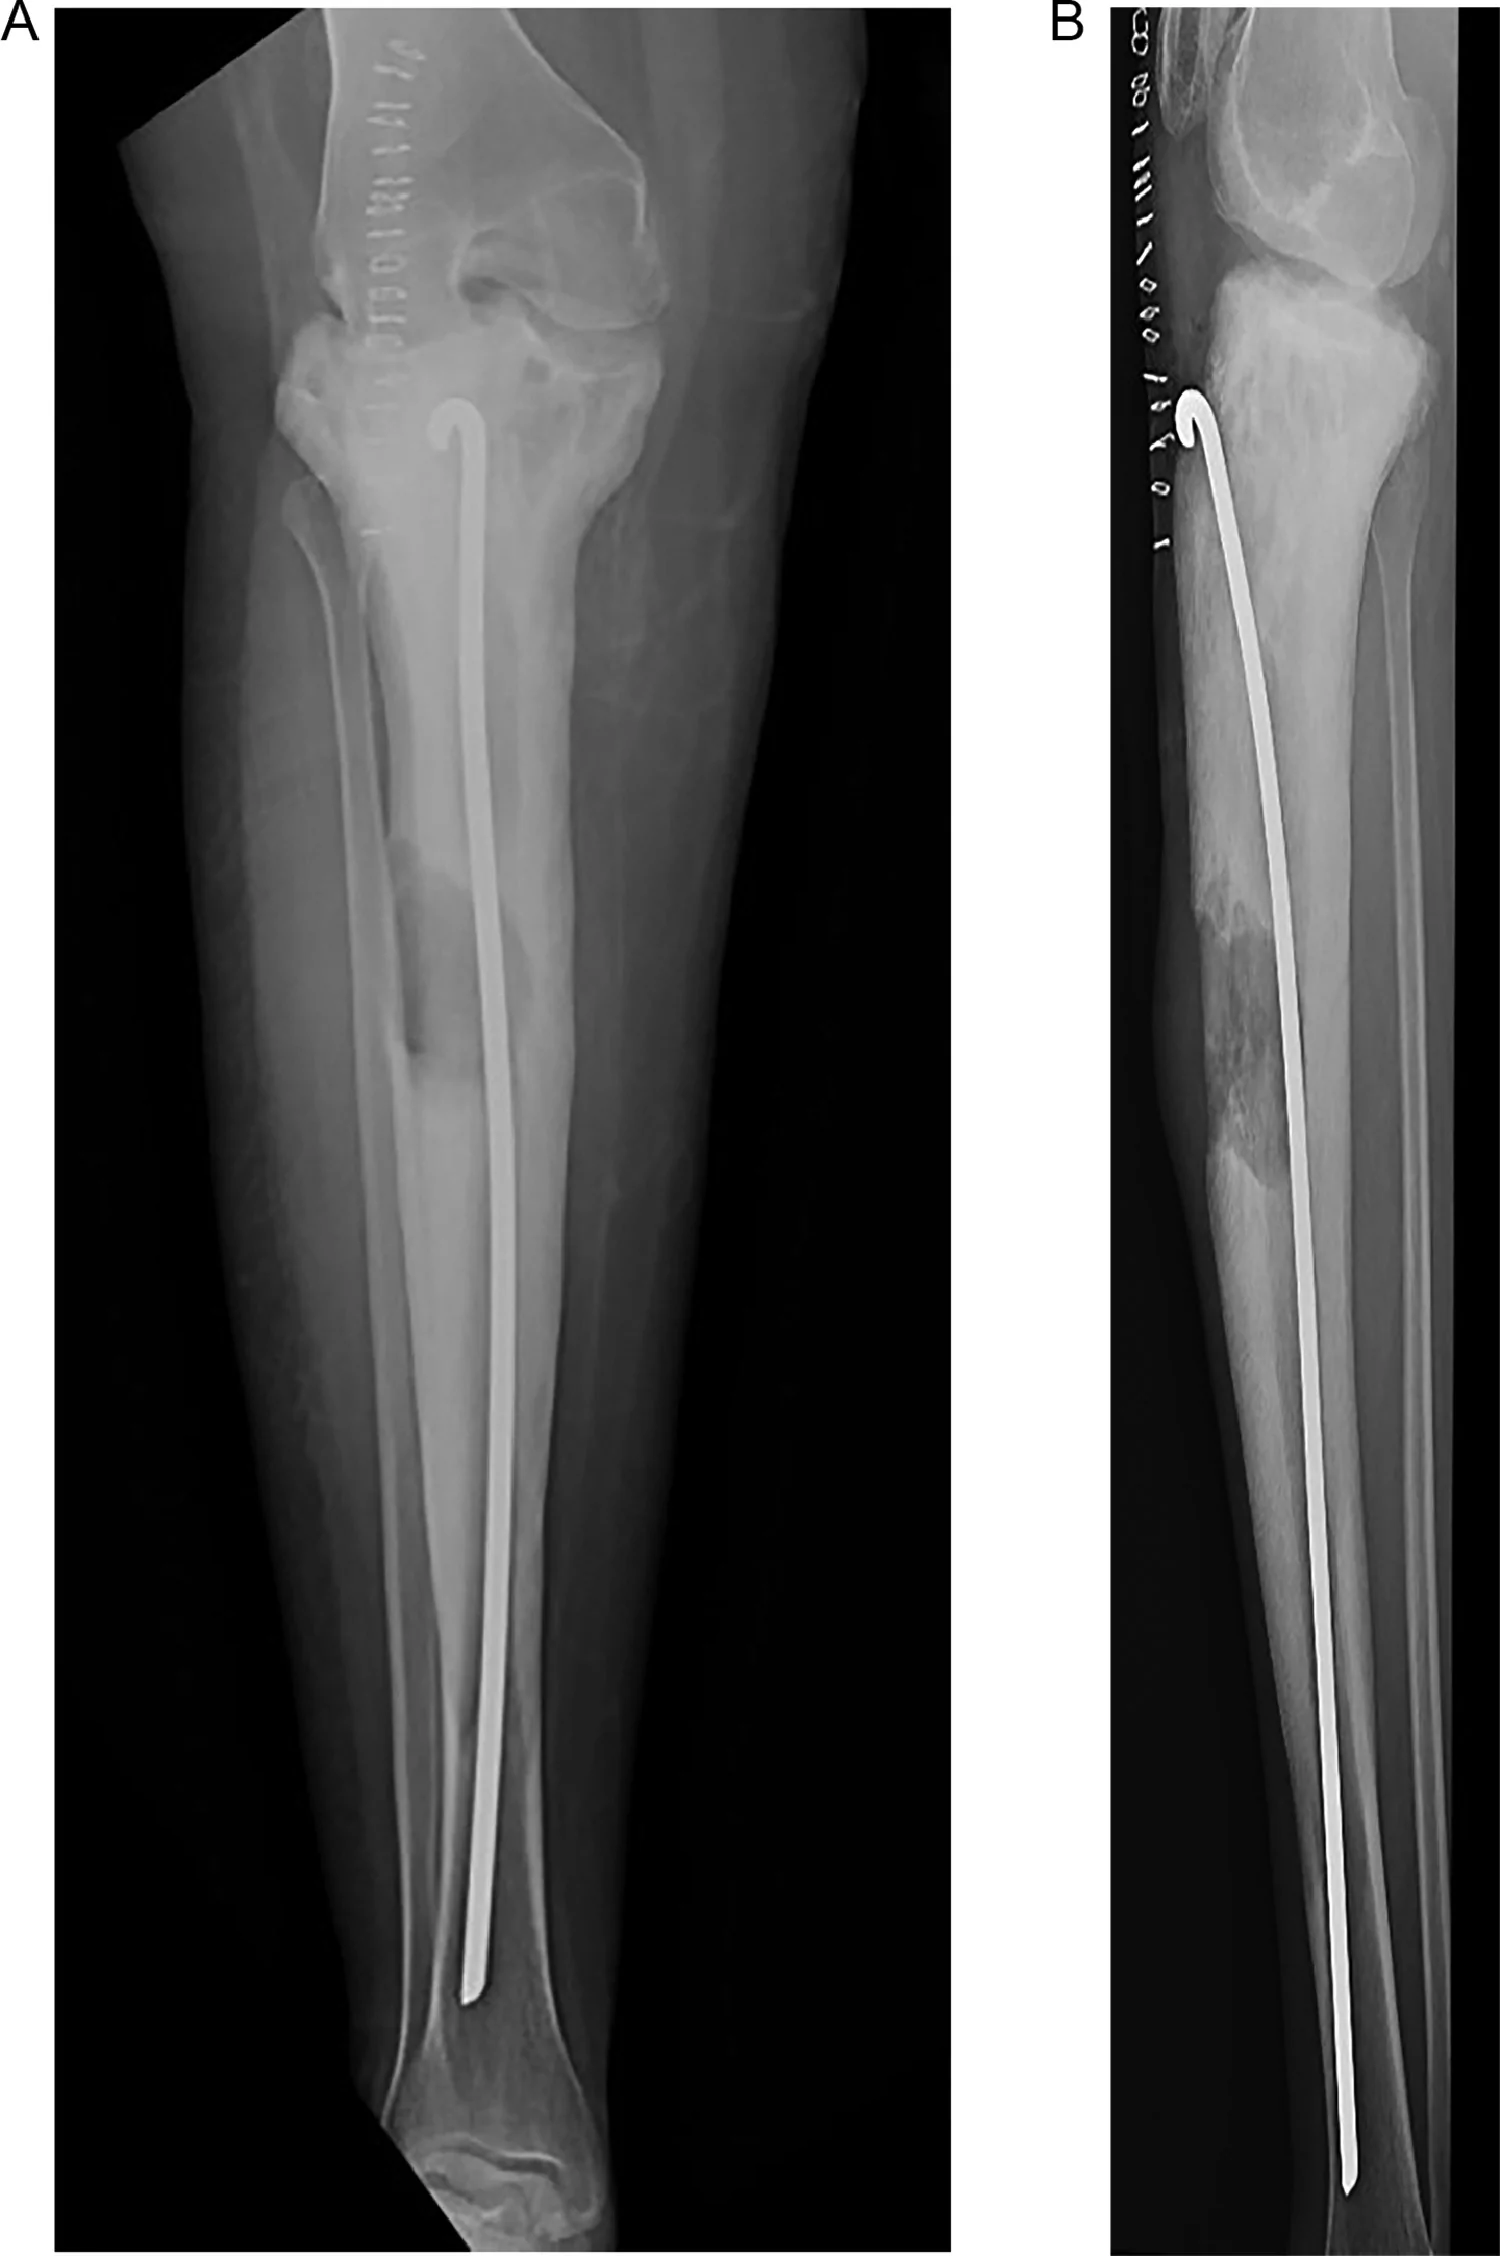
Postoperative AP (A) and lateral (B) radiographs of the right tibia/fibula demonstrate prophylactic intramedullary nailing of the right tibia performed due to the risk of pathologic fracture of the tibial lesion.
